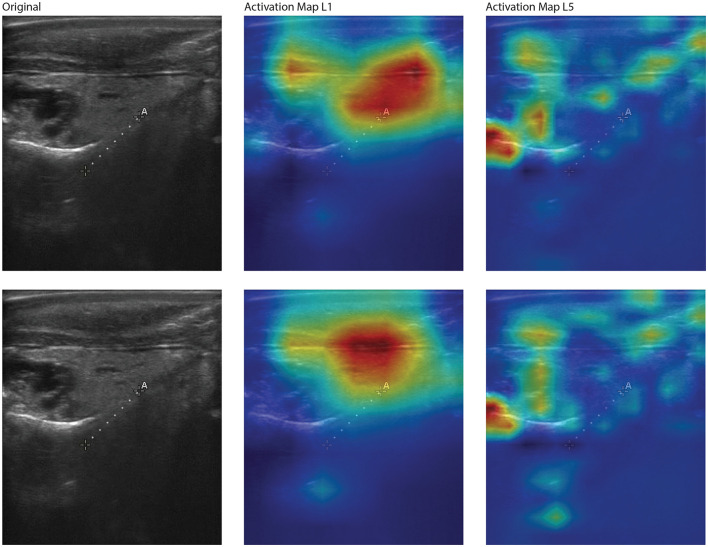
Feature activation maps from the best-performing YOLOv8 model for two correctly classified large male red abalone (*Haliotis rufescens*) images. Each row shows the original ultrasound image (left), the first-layer activation map (L1, middle), and the fifth-layer activation map (L5, right). Activation intensity follows a gradient from dark purple (lowest) through blue, green, and yellow to red (highest). At L1, activation covers a broad region spanning the gonad and extending into adjacent tissue. At L5, activation redistributes into multiple smaller foci, including the lower gonad boundary and areas below the gonad. This pattern is consistent with the female activation maps (Figure 6): early layers respond to large-scale tissue structure while deeper layers resolve spatially distinct anatomical features.
